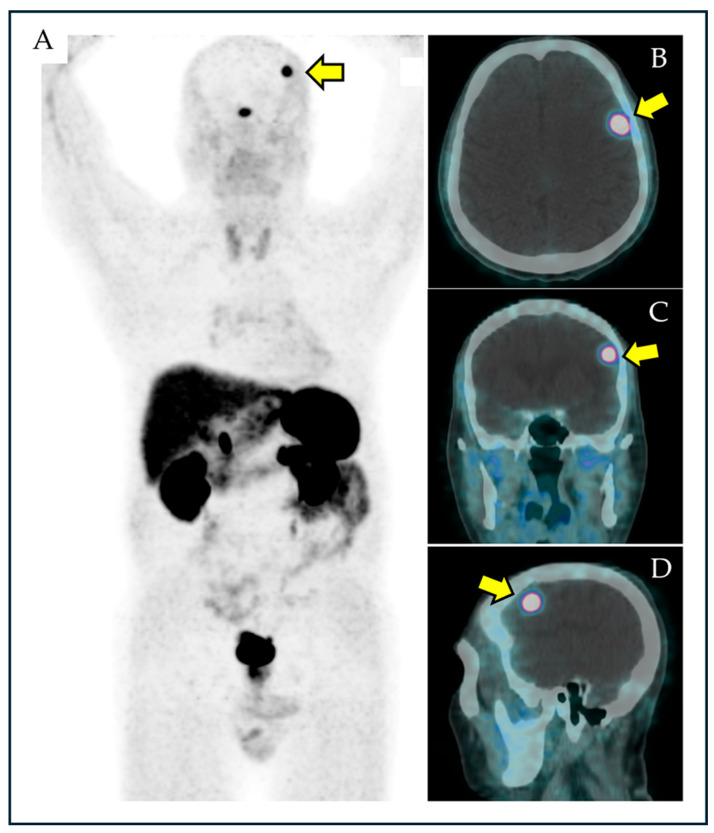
A case of brain incidental finding (yellow arrow) at somatostatin receptor PET/CT with [^68^Ga]Ga-DOTA-peptides detected at maximum-intensity projection PET image (A), axial (B), coronal (C), and sagittal (D) PET/CT images.
